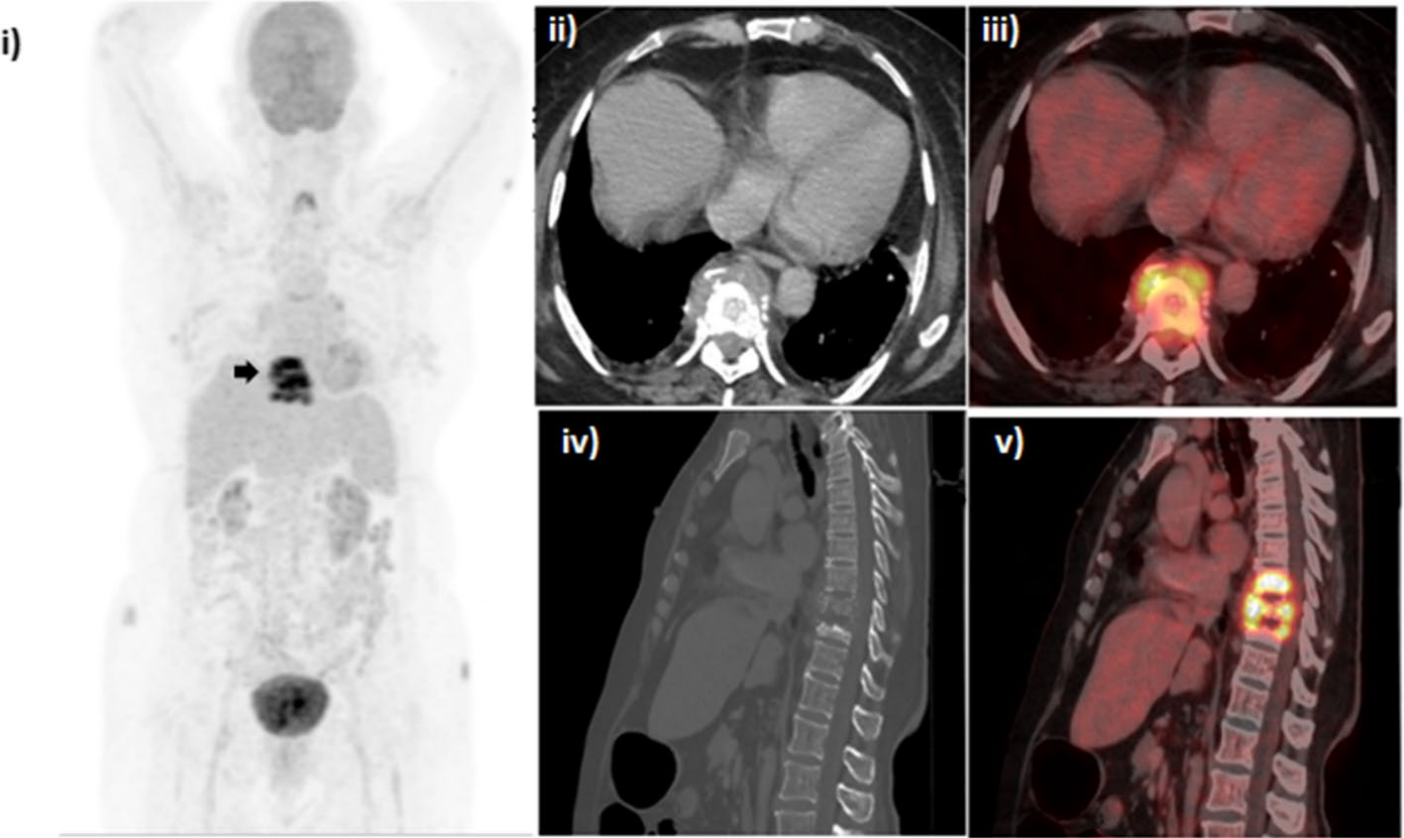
F-18 FDG PET/CT, i MIP images, ii–v CT and fused images. Black arrows show the pathological lesion
